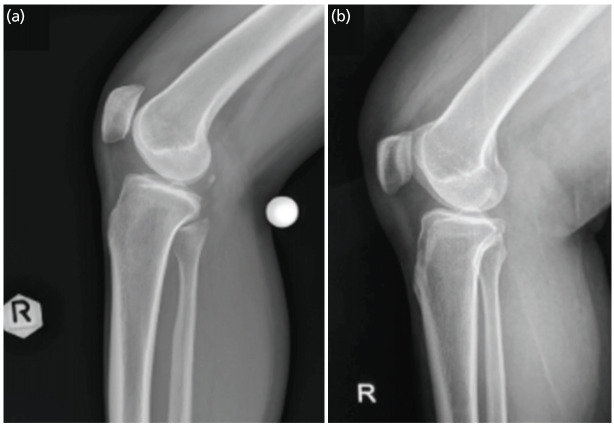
Direct comparison of the lateral knee imaging result from both methods. (a) An example results from the KM method. After the knee-flexion plane was adjusted to become parallel with the image receptor (IR), the femoral condyles were perfectly superimposed with a clear view of patellofemoral joint space, a true lateral knee radiograph. (b) Compared with an example result from the traditional procedure. Since the knee flexion plane was not parallel to the IR, the femoral condyles were not superimposed, and the patellofemoral joint space could not be seen.
